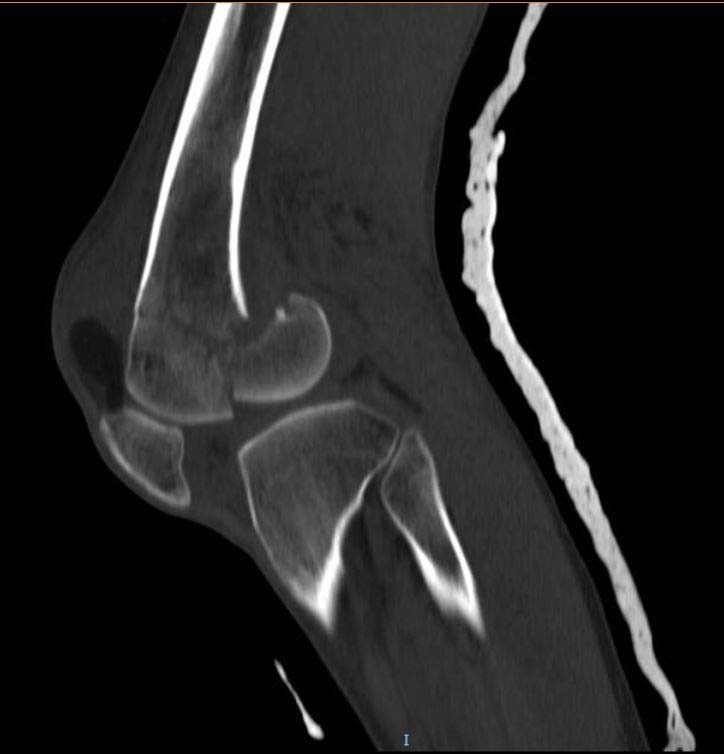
CT Scan in Sagittal Section showing a coronal plane fracture of distal femur (Hoffa Fracture).
